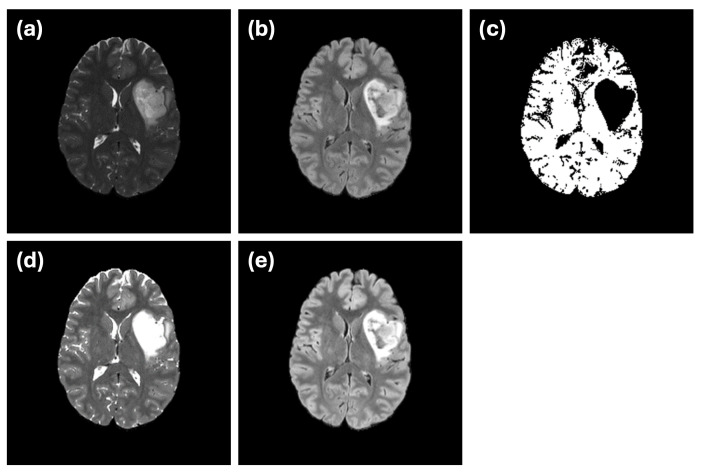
Example of parenchyama-based normalization. (a) T2-w and (b) FLAIR images are normalized to (d) and (e), respectively, using parenchyama label in (c).
